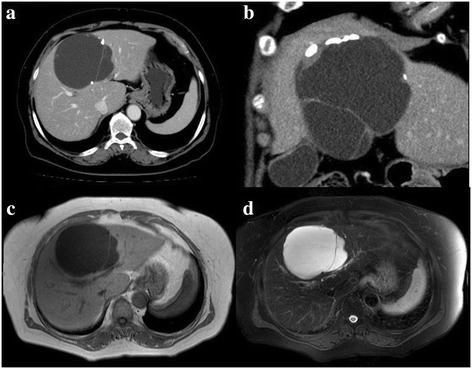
CT and T1- and T2-weighted images. CT showing a multilocular cystic lesion in the medial segment of the liver with calcification in the cyst wall (a). Thickening of the cyst wall around the calcification (b). Contrast-enhanced CT showed no enhancement of the thickened wall (a, b). The cystic lesion was visualized as a hypointensity on T1-weighted images (c) and as a hyperintensity on the T2-weighted images (d).
